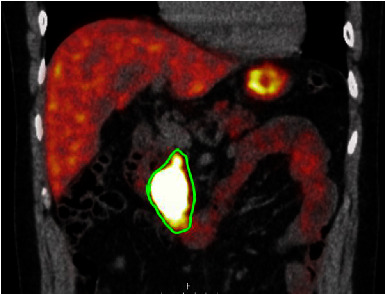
PET-CT 68Ga-DOTATATE scan showing a 3 cm lesion in the head of the pancreas (green outline).
